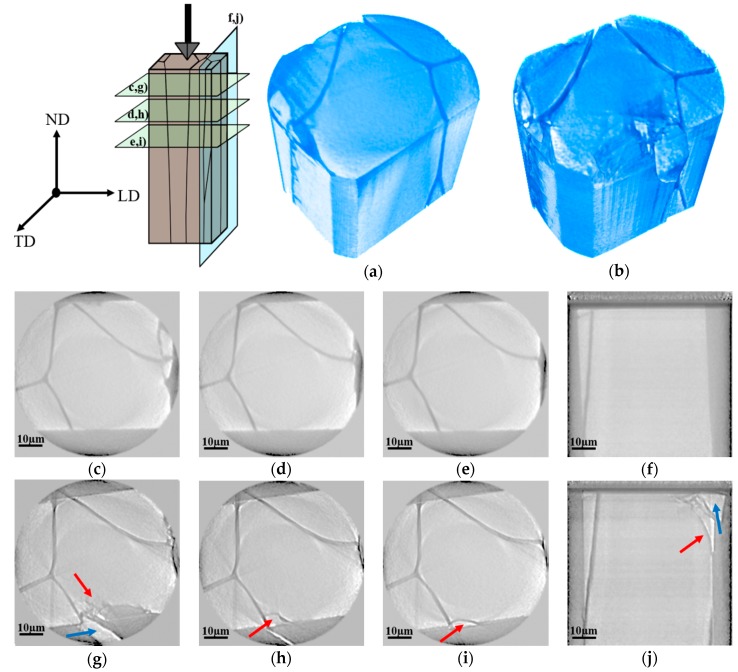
Scheme of in-situ X-Ray Computed Tomography indentation of *P. margaritifera* shells along ND, 3D reconstruction of the sample before (a) and after (b) in-situ indentation test; horizontal and vertical virtual cross-sections of the shell acquired before (c–f) and after (g–j) the indentation test (red arrows point to the cracks, while blue arrows show part of the material damaged during the test).
